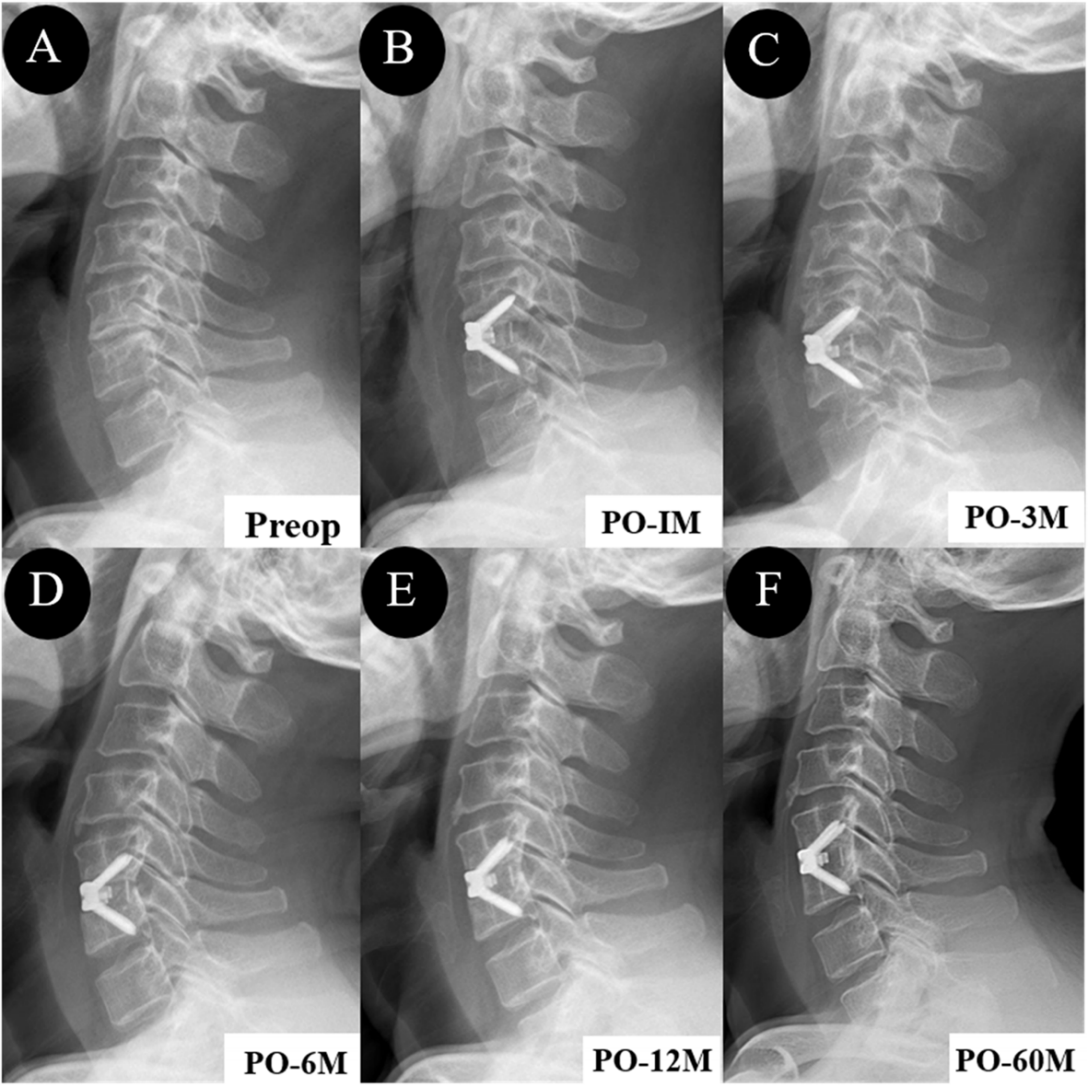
Serial lateral X-ray images of a 54-year-old man who underwent single-level ACDF surgery at C5-C6. (A) Preoperative lateral X-ray image. (B-F) Postoperative lateral X-ray images obtained at immediately (< 1 week), 3 months, 6 months, 12 months, and 60 months show satisfactory cervical sagittal alignment
